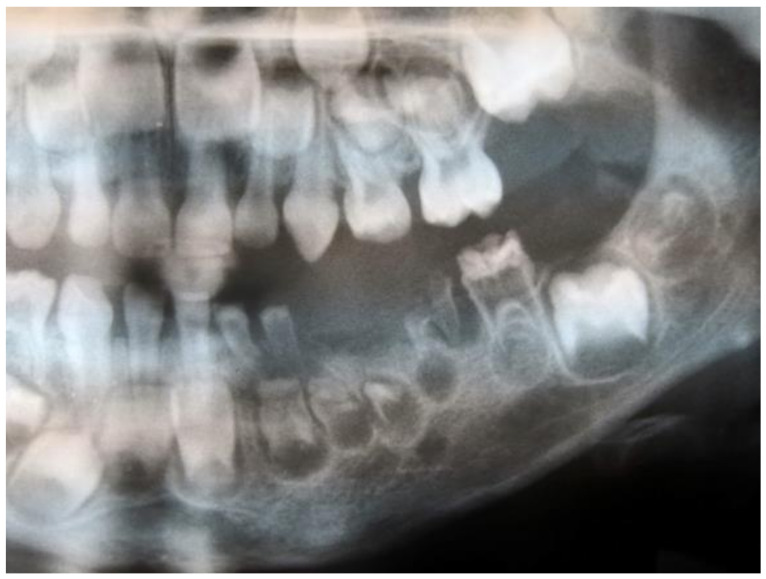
Fragment of the panoramic radiograph showing “ghost teeth” (in the mandibular left quadrant) characteristic of regional odontodysplasia—personal collection (K.N., Poland, 2019).
